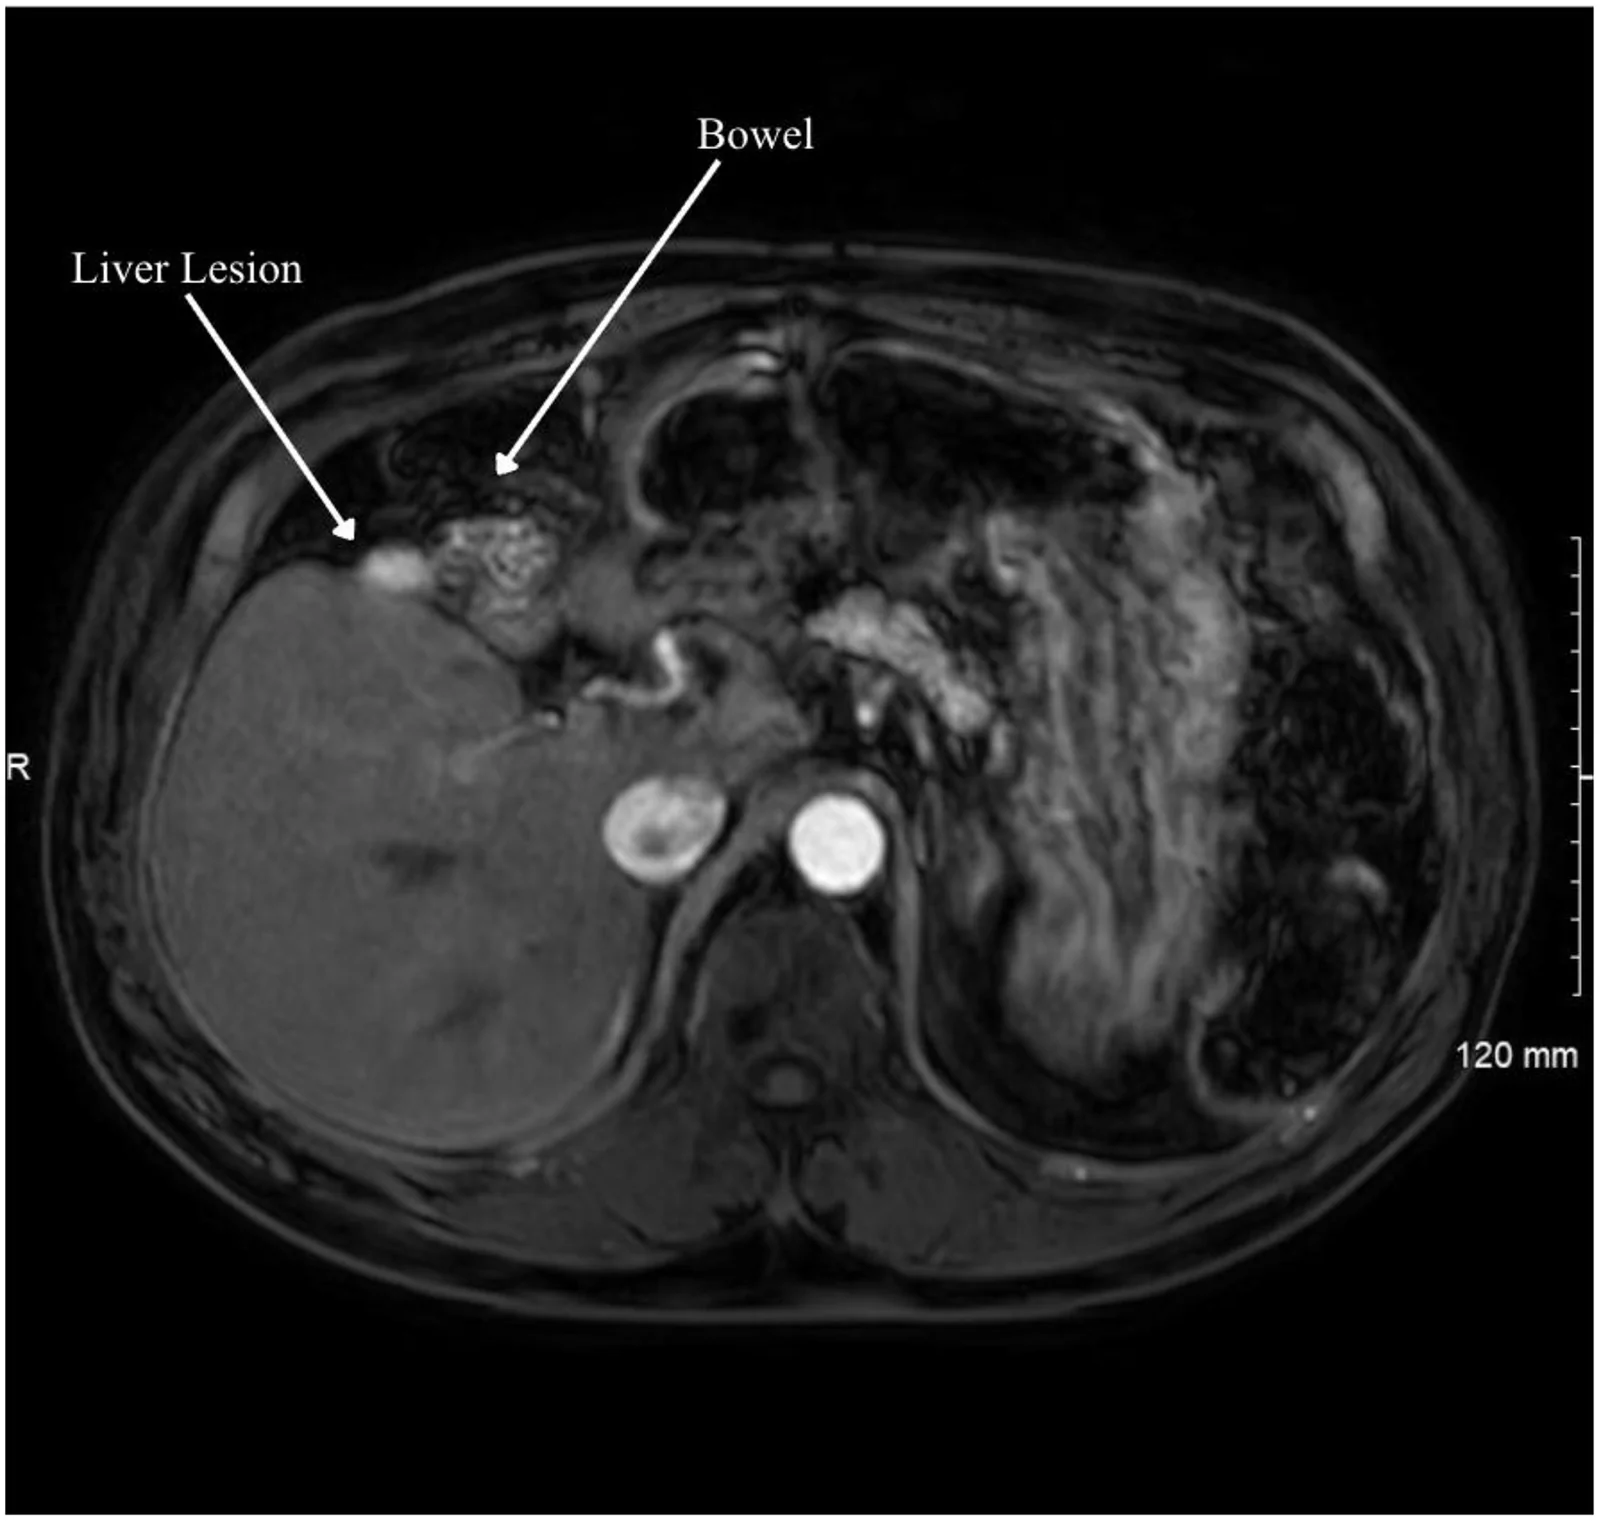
T1-weighted MRI abdomen demonstrating postcontrast arterial enhancement of a subcapsular exophytic hepatic lesion, with immediately adjacent large bowel.
